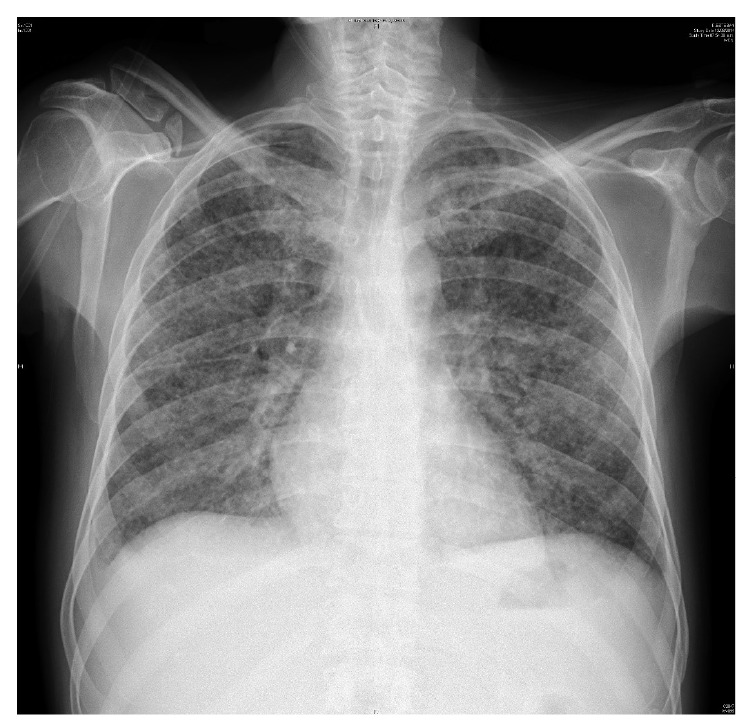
Chest x-ray showing bilateral diffuse interstitial infiltrates
